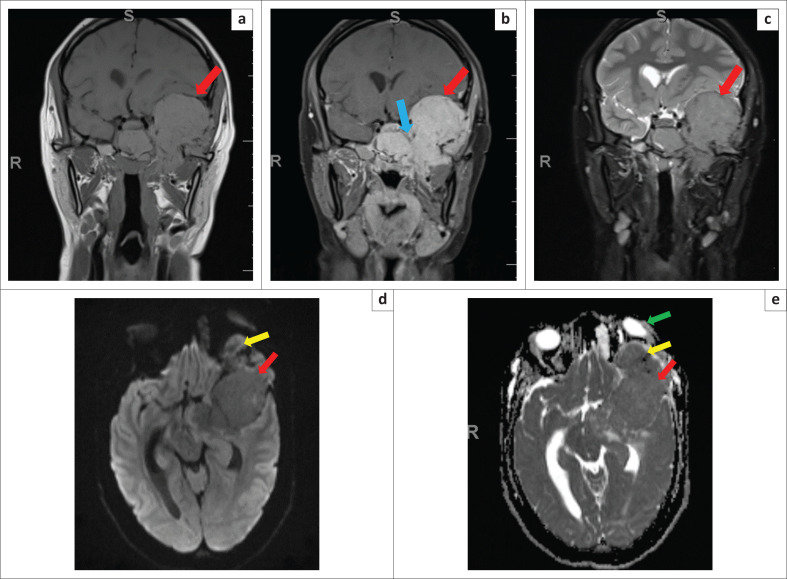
(a–c) Coronal pre-contrast T1-weighted (T1W), post contrast T1W fat saturation (FS) and T2-weighted (T2W) FS at the same level. The trans-spatial base of skull tumour (red arrow) is hyperintense to muscle on T1W (a) and T2W FS (c) and avidly enhances on post contrast T1W FS (b). Invasion of the left cavernous sinus is demonstrated (blue arrow). (d–e) No restricted diffusion (red arrow) on axial diffusion-weighted imaging (DWI) (d) and apparent diffusion coefficient (ADC) (e). The tumour is shown to extend into the extraconal compartment of the left orbit (yellow arrow) with resultant proptosis of the left globe (green arrow).
